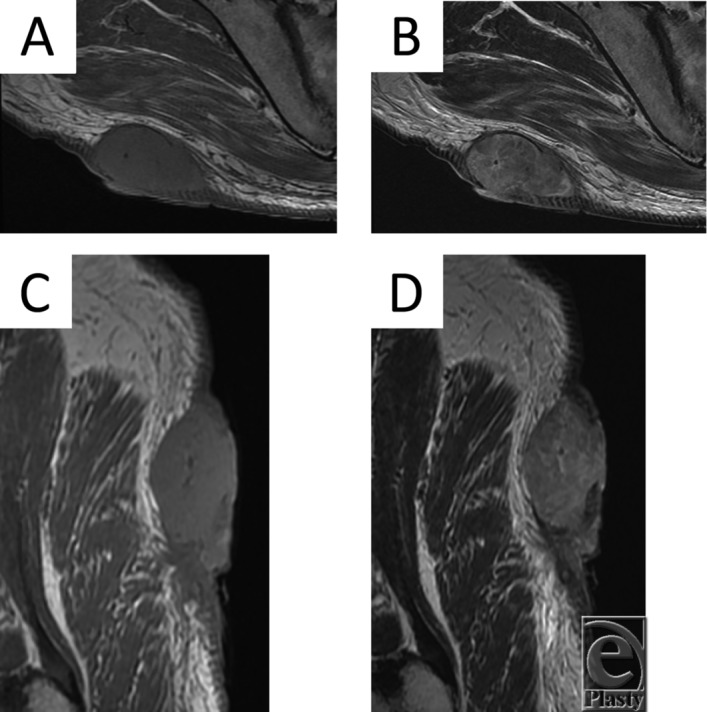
(*a*) Axial T1-weighted MRI scan of the tumor. (*b*) Axial T2-weighted MRI scan of the tumor. (*c*) Sagittal T1-weighted MRI scan of the tumor. (*d*) Sagittal T2-weighted MRI scan of the tumor.
